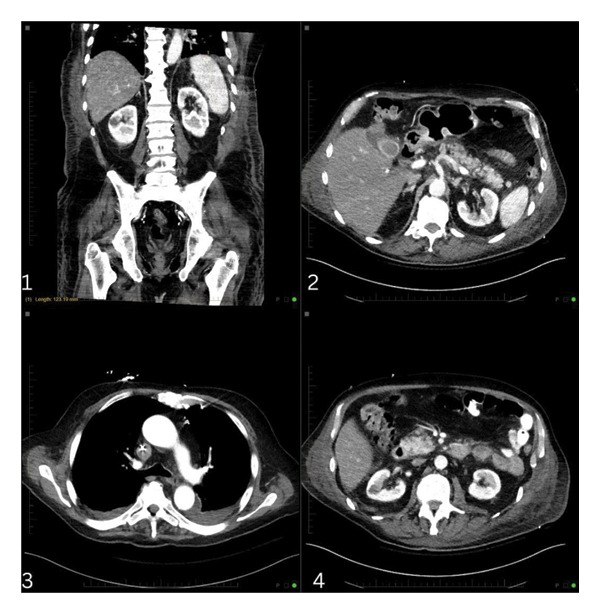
CT chest, abdomen, and pelvis with contrast: showed (image 1) resolution of splenomegaly (now 12.3 cm), (image 2) complete resolution of 4 cm nodular density within the pancreatic tail, (image 3) resolution of extensive lymphadenopathy, and (image 4) resolution of periaortic lymphadenopathy.
